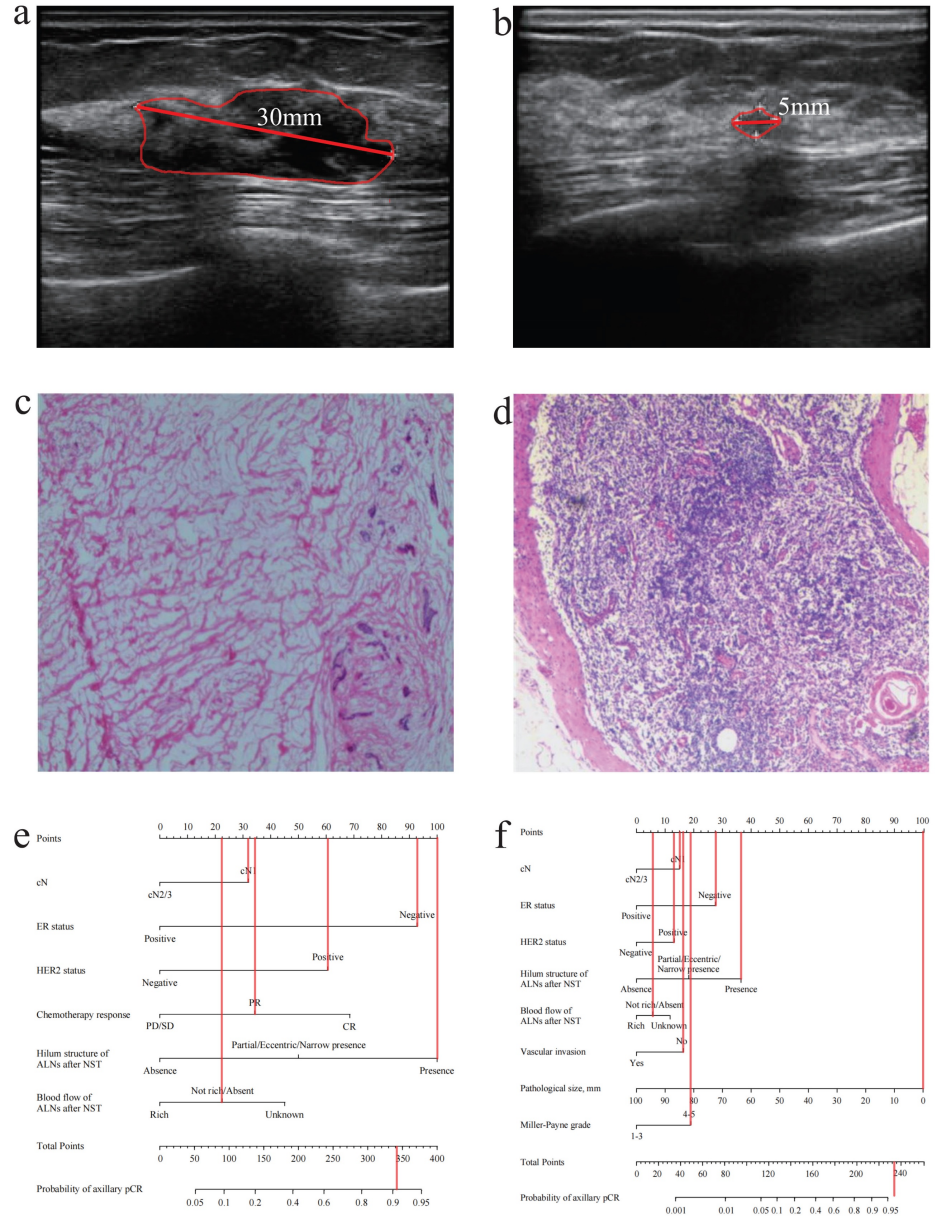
A patient with axillary pCR (cN1, negative ER status, positive HER2 status, present hilum structure of ALNs after NST, absent blood flow of ALNs after NST, no vascular invasion, pathological size: 0mm, Miller-Payne grade: 5). (a) The ultrasound before NST (the diameter of tumor: 30mm). (b) The ultrasound after NST (the diameter of tumor: 5mm). (c) The pathology of breast after operation (breast pCR). (d) The pathology of ALNs after NST (axillary pCR). (e) The probability of axillary pCR was approximately 90% based on the US-based model. (f) The probability of axillary pCR was over 95% based on the combined model. pCR, pathological complete response; cN, clinical lymph nodes; ALNs, axillary lymph nodes; NST, neoadjuvant systemic therapy; US, ultrasound.
